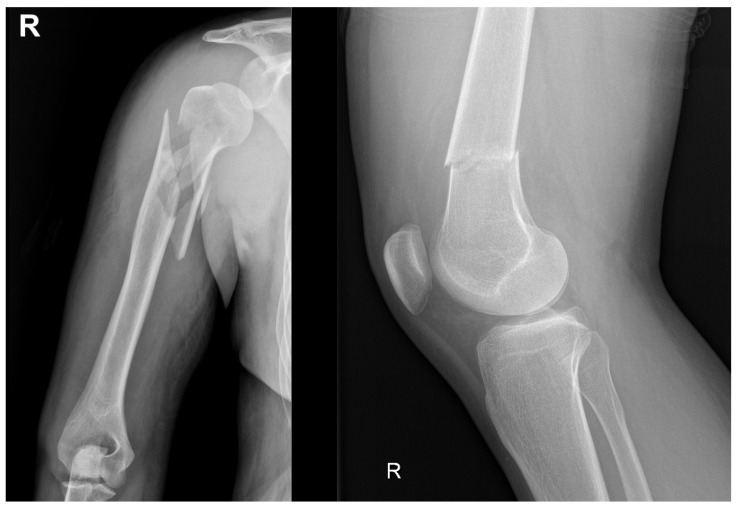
The (left) image shows a comminuted (multiple fragments) proximal humerus fracture. The (right) radiograph shows a transverse fracture of the distal femur. This is a patient from one of the authors (J.P.M.-C.): office at Fundación Valle del Lili, Cali, Colombia, with a signed consent form.
